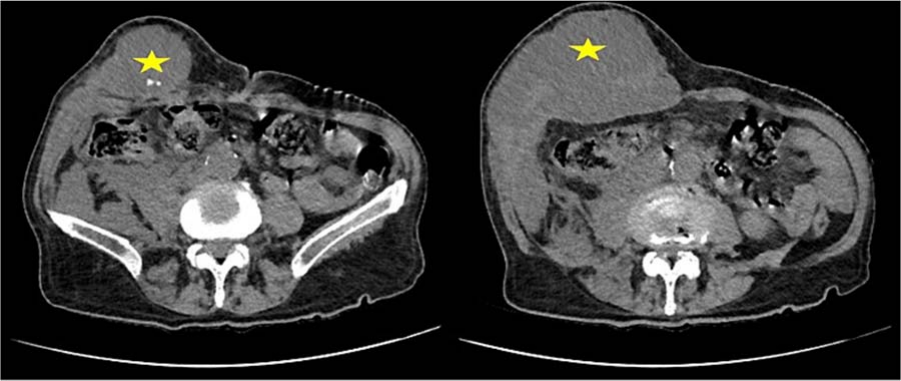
Contrast-enhanced CT scan of the abdominal wall showing a parietal abscess containing hyperdense foci consistent with retained gallstones (star).
